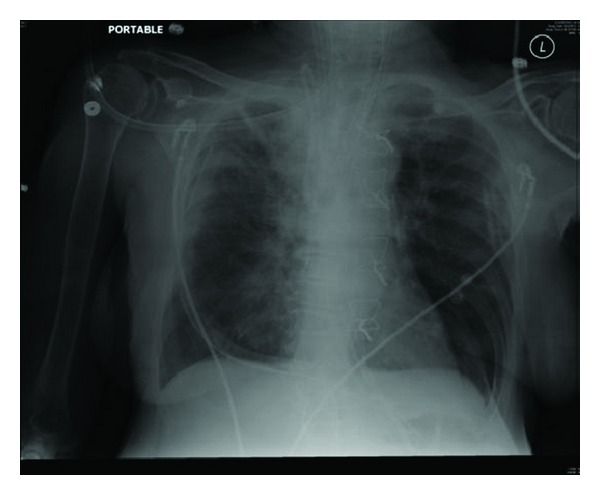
Postoperative chest X-ray: significant improvement of the right mediastinal hemothorax.
